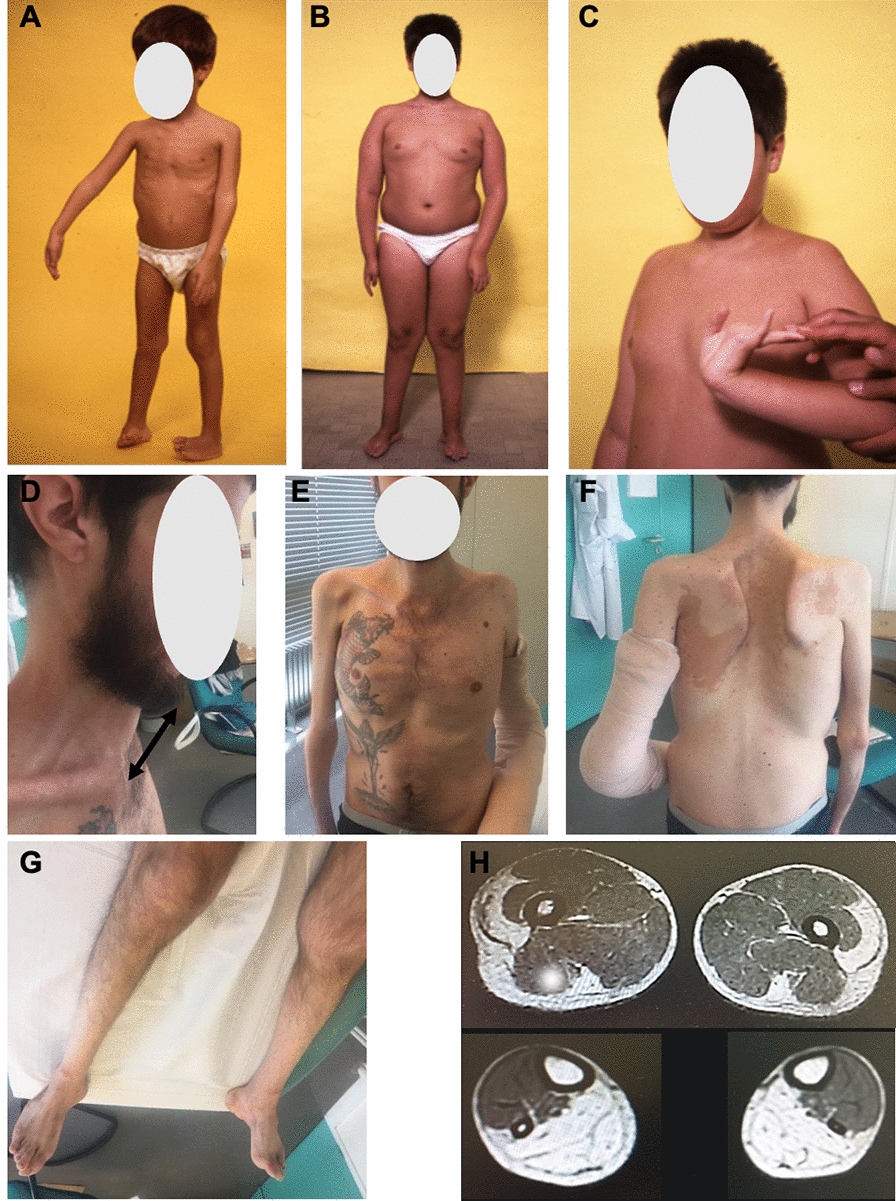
Clinical and imaging features of P1 at 4, 8 and 33 years old. A P1 at 4 years. Note the thin muscle bulk, the proximo-distal weakness and atrophy. B P1 at 8 years showing elbow contractures, and leg atrophies. C Hyperlaxity of wrist joint. D Cervical rigid spine. E Thoracic deformation and amyotrophy. F Scapular winging associated with scoliosis. G Prominent distal atrophy. H Muscle MRI at thigh and leg level showing bilateral and symmetric patchy adipose replacement of vastus lateralis and semitendinosus muscles, and complete replacement of posterior leg compartment
